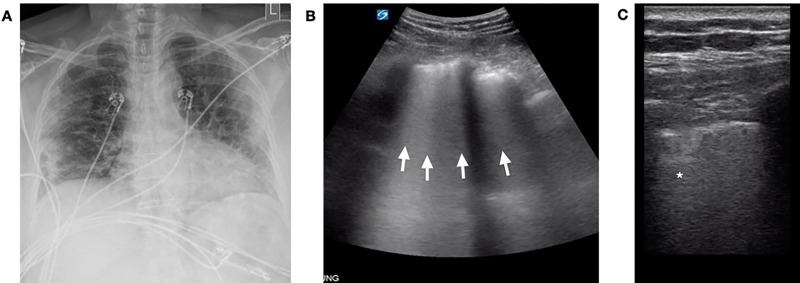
Case 2 imaging findings: (A) Anterior-posterior chest radiograph notable for peripherally distributed bilateral patchy opacities. Lung point-of-care ultrasound was notable for diffuse B-lines coalescing to involve the entire rib space (arrows) laterally (B), while posterior views revealed irregular pleural lines and subpleural consolidations (asterisk, C).
